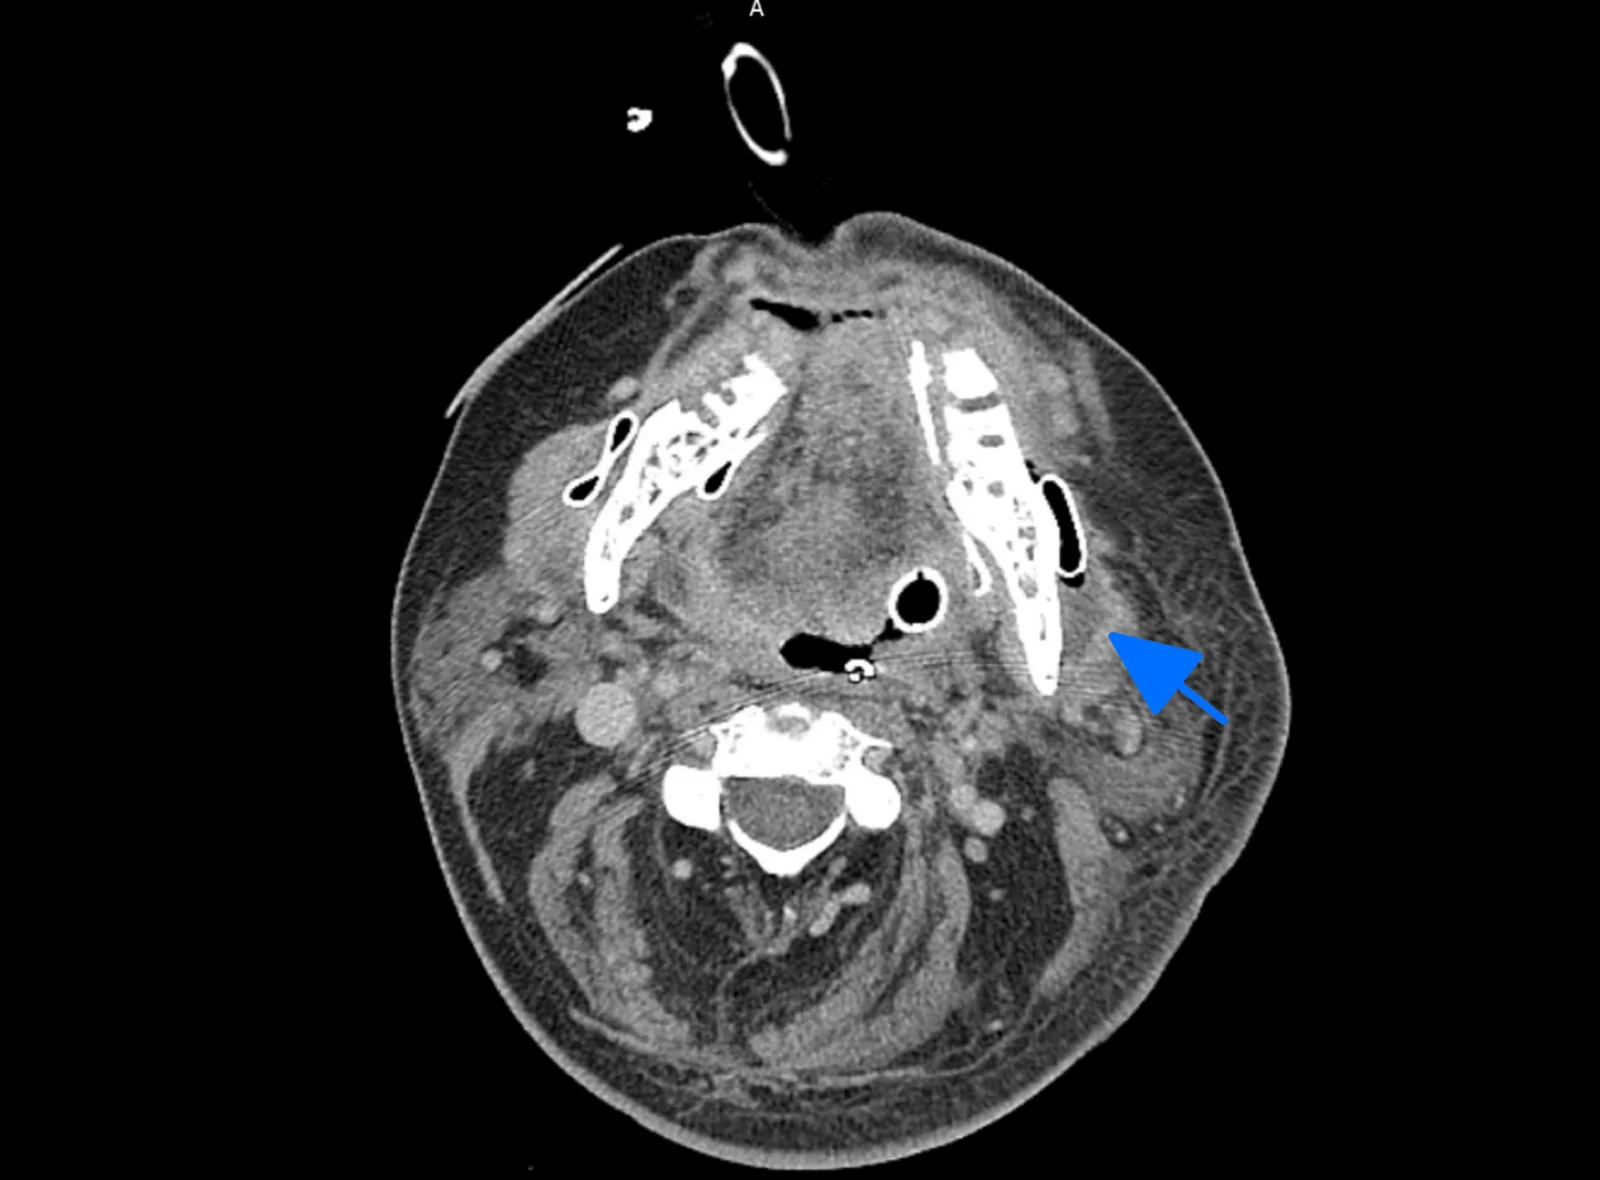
Post-operative axial contrast CT of the maxillofacial showing a right parasymphysis fracture with a remnant abscess in the left masticator space (blue arrow).
